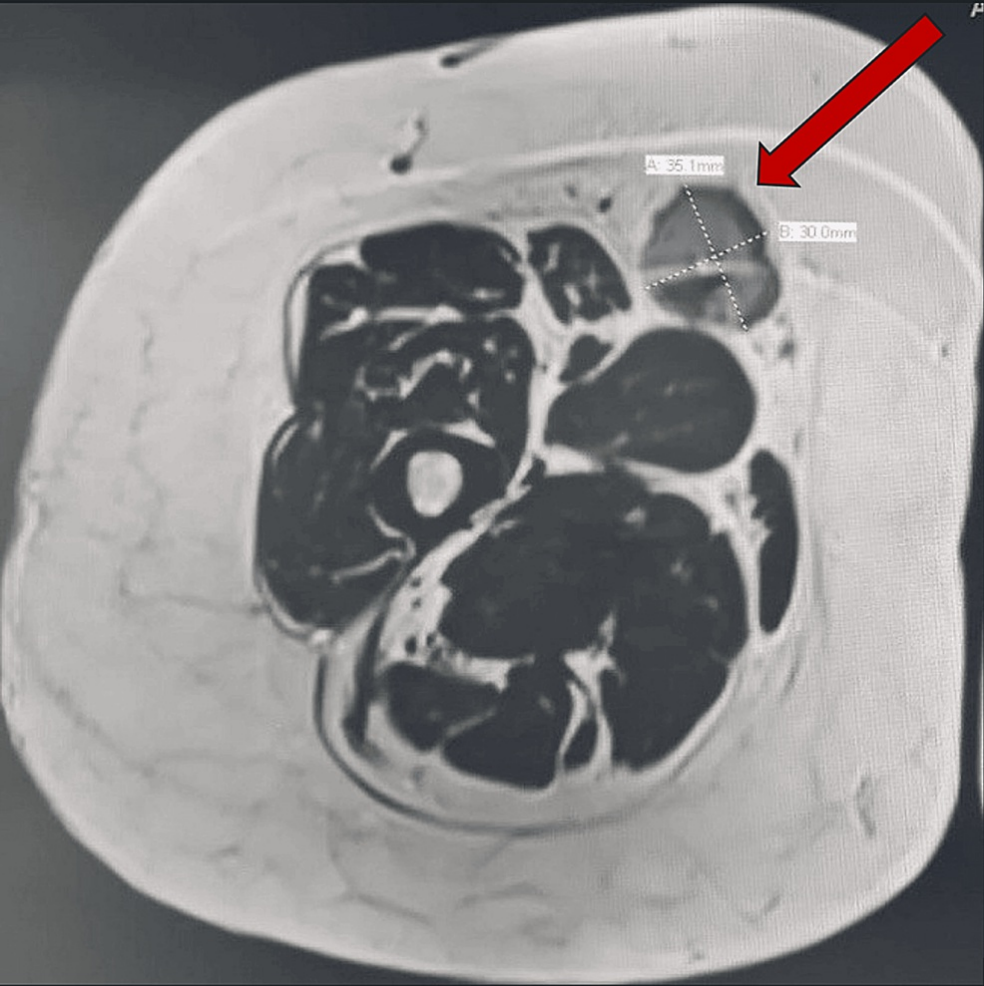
MRI showing a mass at the anterolateral right thigh
